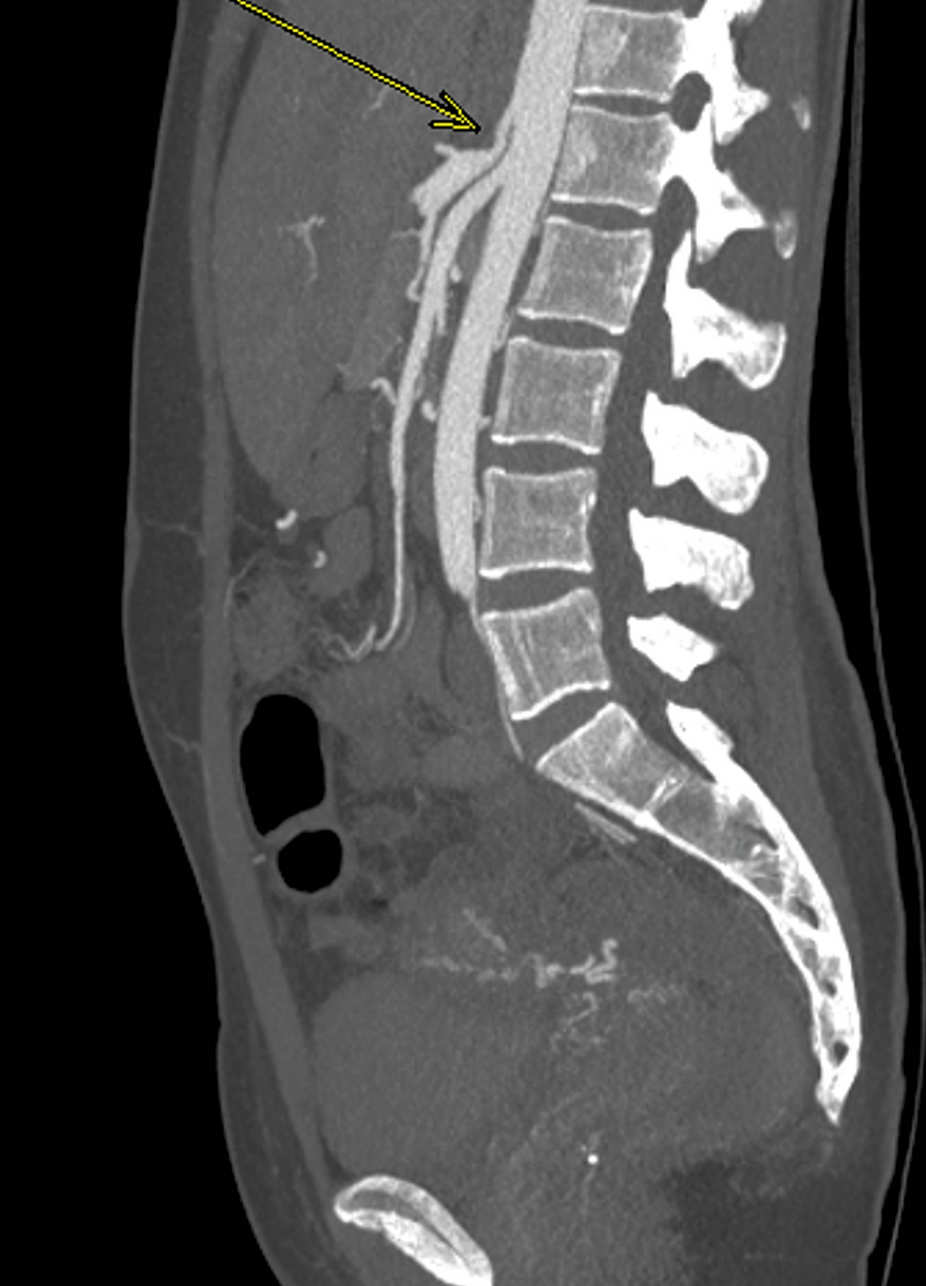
CTA showing compression of the celiac trunk as indicated by the arrow. CTA: computed tomography angiogram
